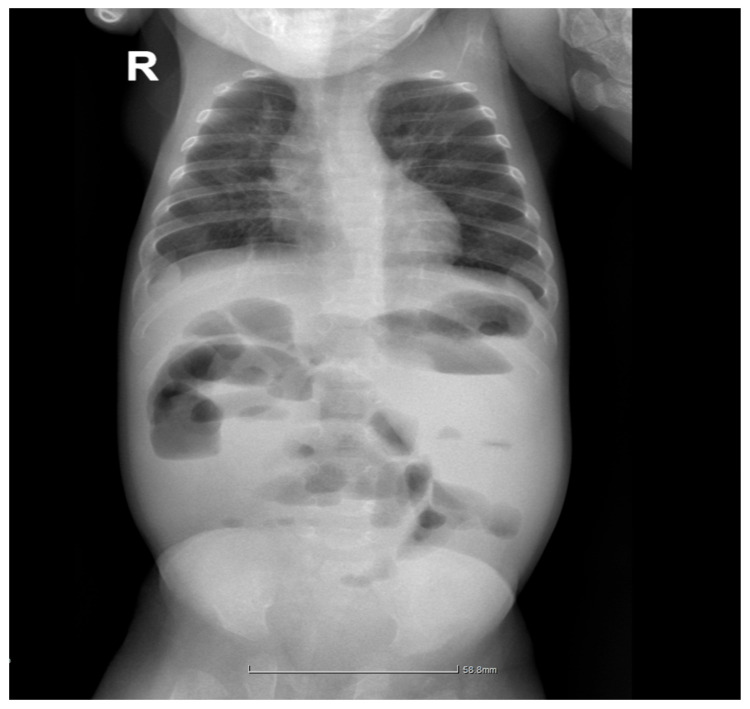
Preoperative abdominal X-ray showing dilated bowel loops and air–fluid levels in the ascending and transverse colon, indicating colostomy dysfunction. These findings necessitated surgical intervention with colostomy closure and end-to-end colo-colic anastomosis (R = right).
